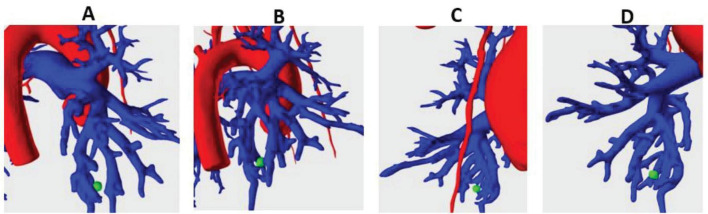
Cohort-level angiographic heterogeneity and 3D-CTA component visualization in inflammatory peripheral PAP. (A–D) Represents 3D-CTA visualization demonstrating component separation for reconstruction and tracing, with the pulmonary arterial tree displayed independently (and, when present, candidate systemic arterial components shown separately) to facilitate subsequent branch-level supply-vessel localization and interventional cross-validation.
